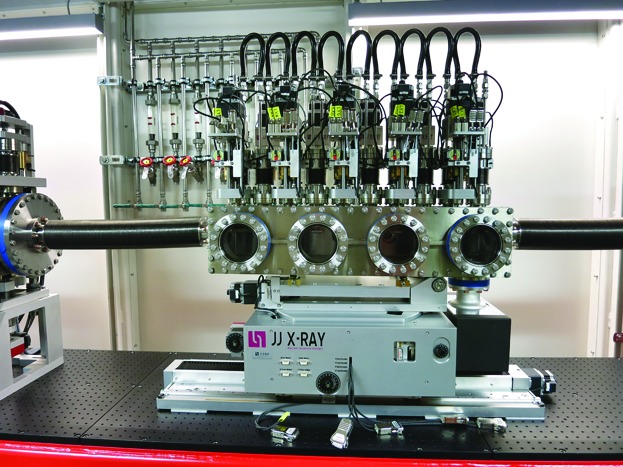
The ten-axis Be-lens chamber allows one to focus all X-ray energies onto the sample 5 m downstream. A chamber translation stage further adjusts the spot sizes within its stroke of ±50 cm.
